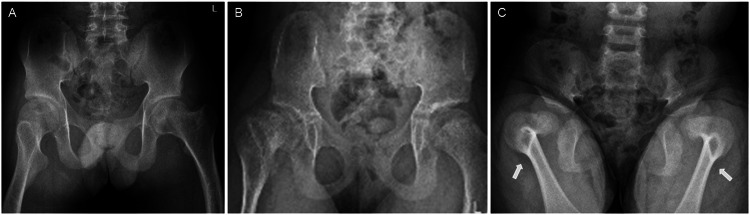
Kyphomelic femora in the participants. Radiographs of pelvis [proband 1 at 15 years (A), proband 2 at 11 years (B) and proband 3 at 3 years of age (C)] show short and broad pelvis, coxa vara and reduced hip joint space in all. Kyphotic femora are seen in all of them but is more prominent (arrows) in the younger proband 3 (C).
